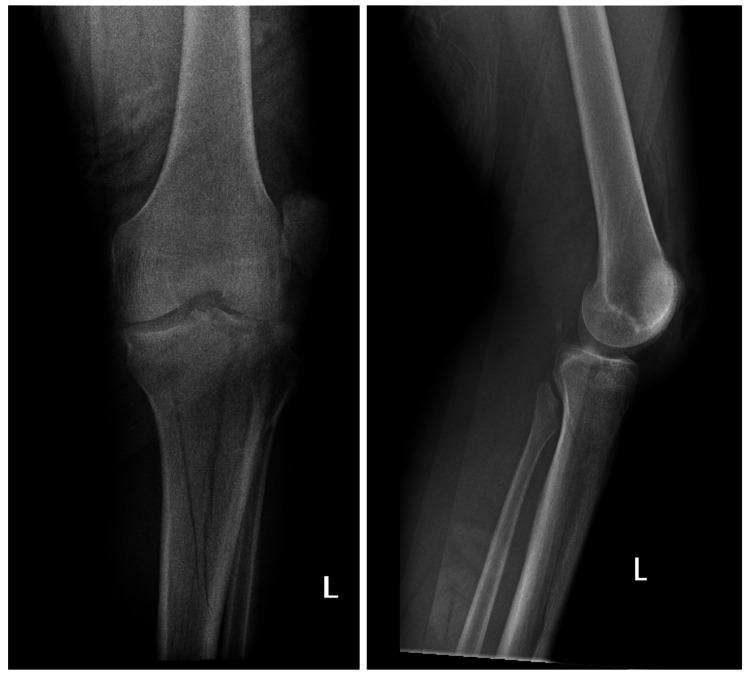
AP and lateral views of the left knee X-ray showing a bicondylar tibial plateau fracture and lateral patella dislocation
